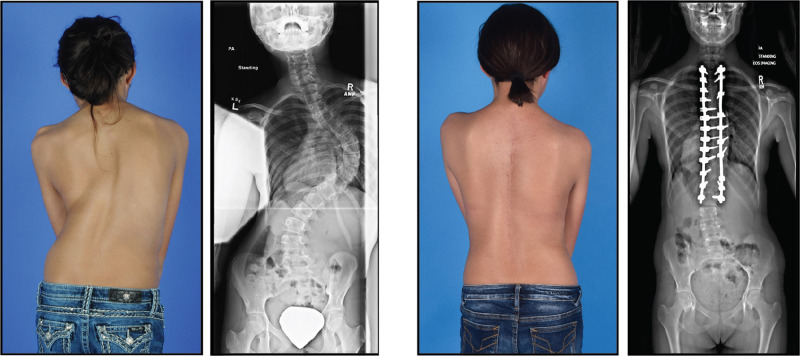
Adolescent idiopathic scoliosis in a female patient. Photograph and standing radiograph (left) reveal a typical right-sided thoracic curve and postural imbalance that is largely corrected with surgical implants (right)
